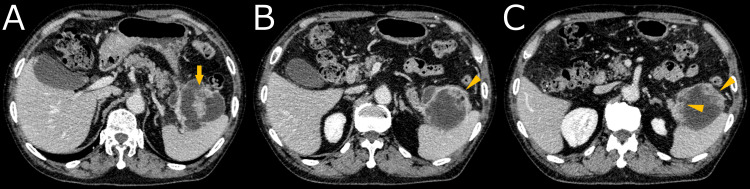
Contrast-enhanced computed tomography A cystic mass protruding from the tail of the pancreas, with enhanced solid components (arrow in A) and irregular thickening of cyst wall (arrowhead in B, C).
